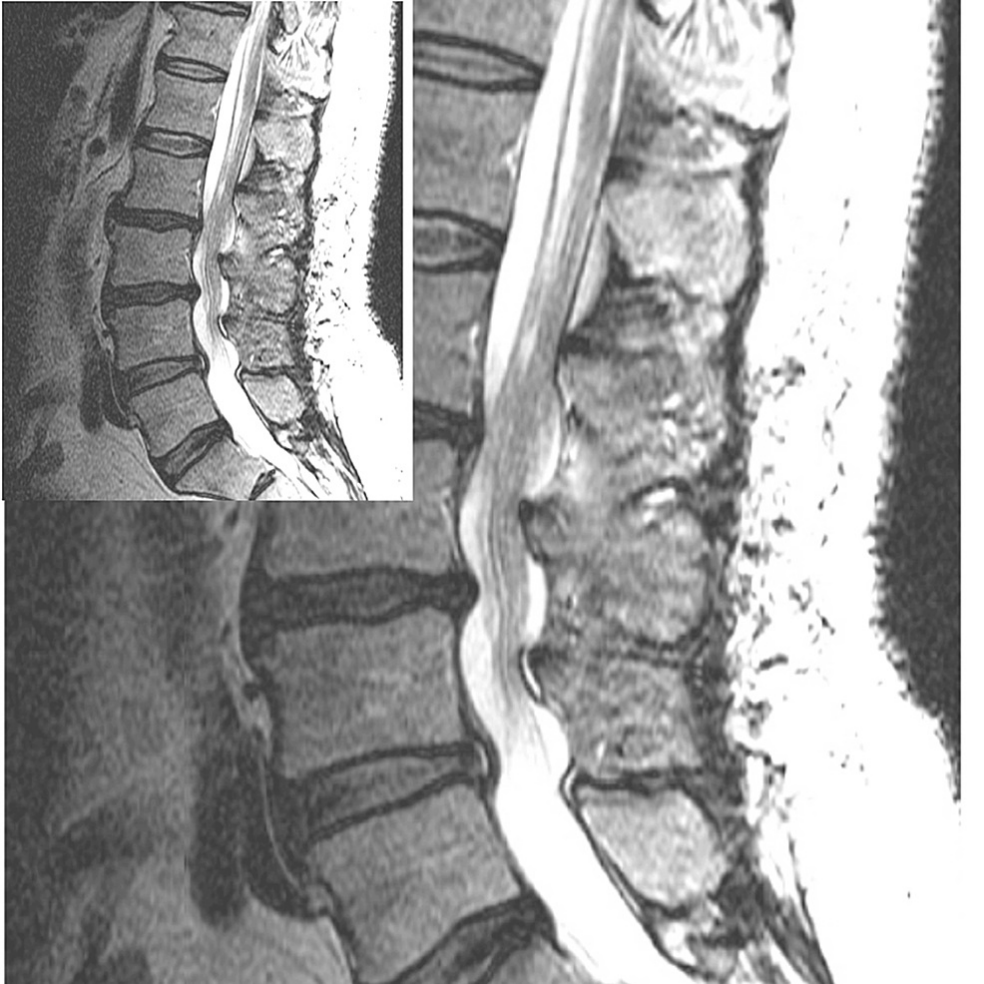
Postoperative T2-weighted MRI of the lumbar spine demonstrating no compressive elements (hematoma or seroma) on the spinal cord.
